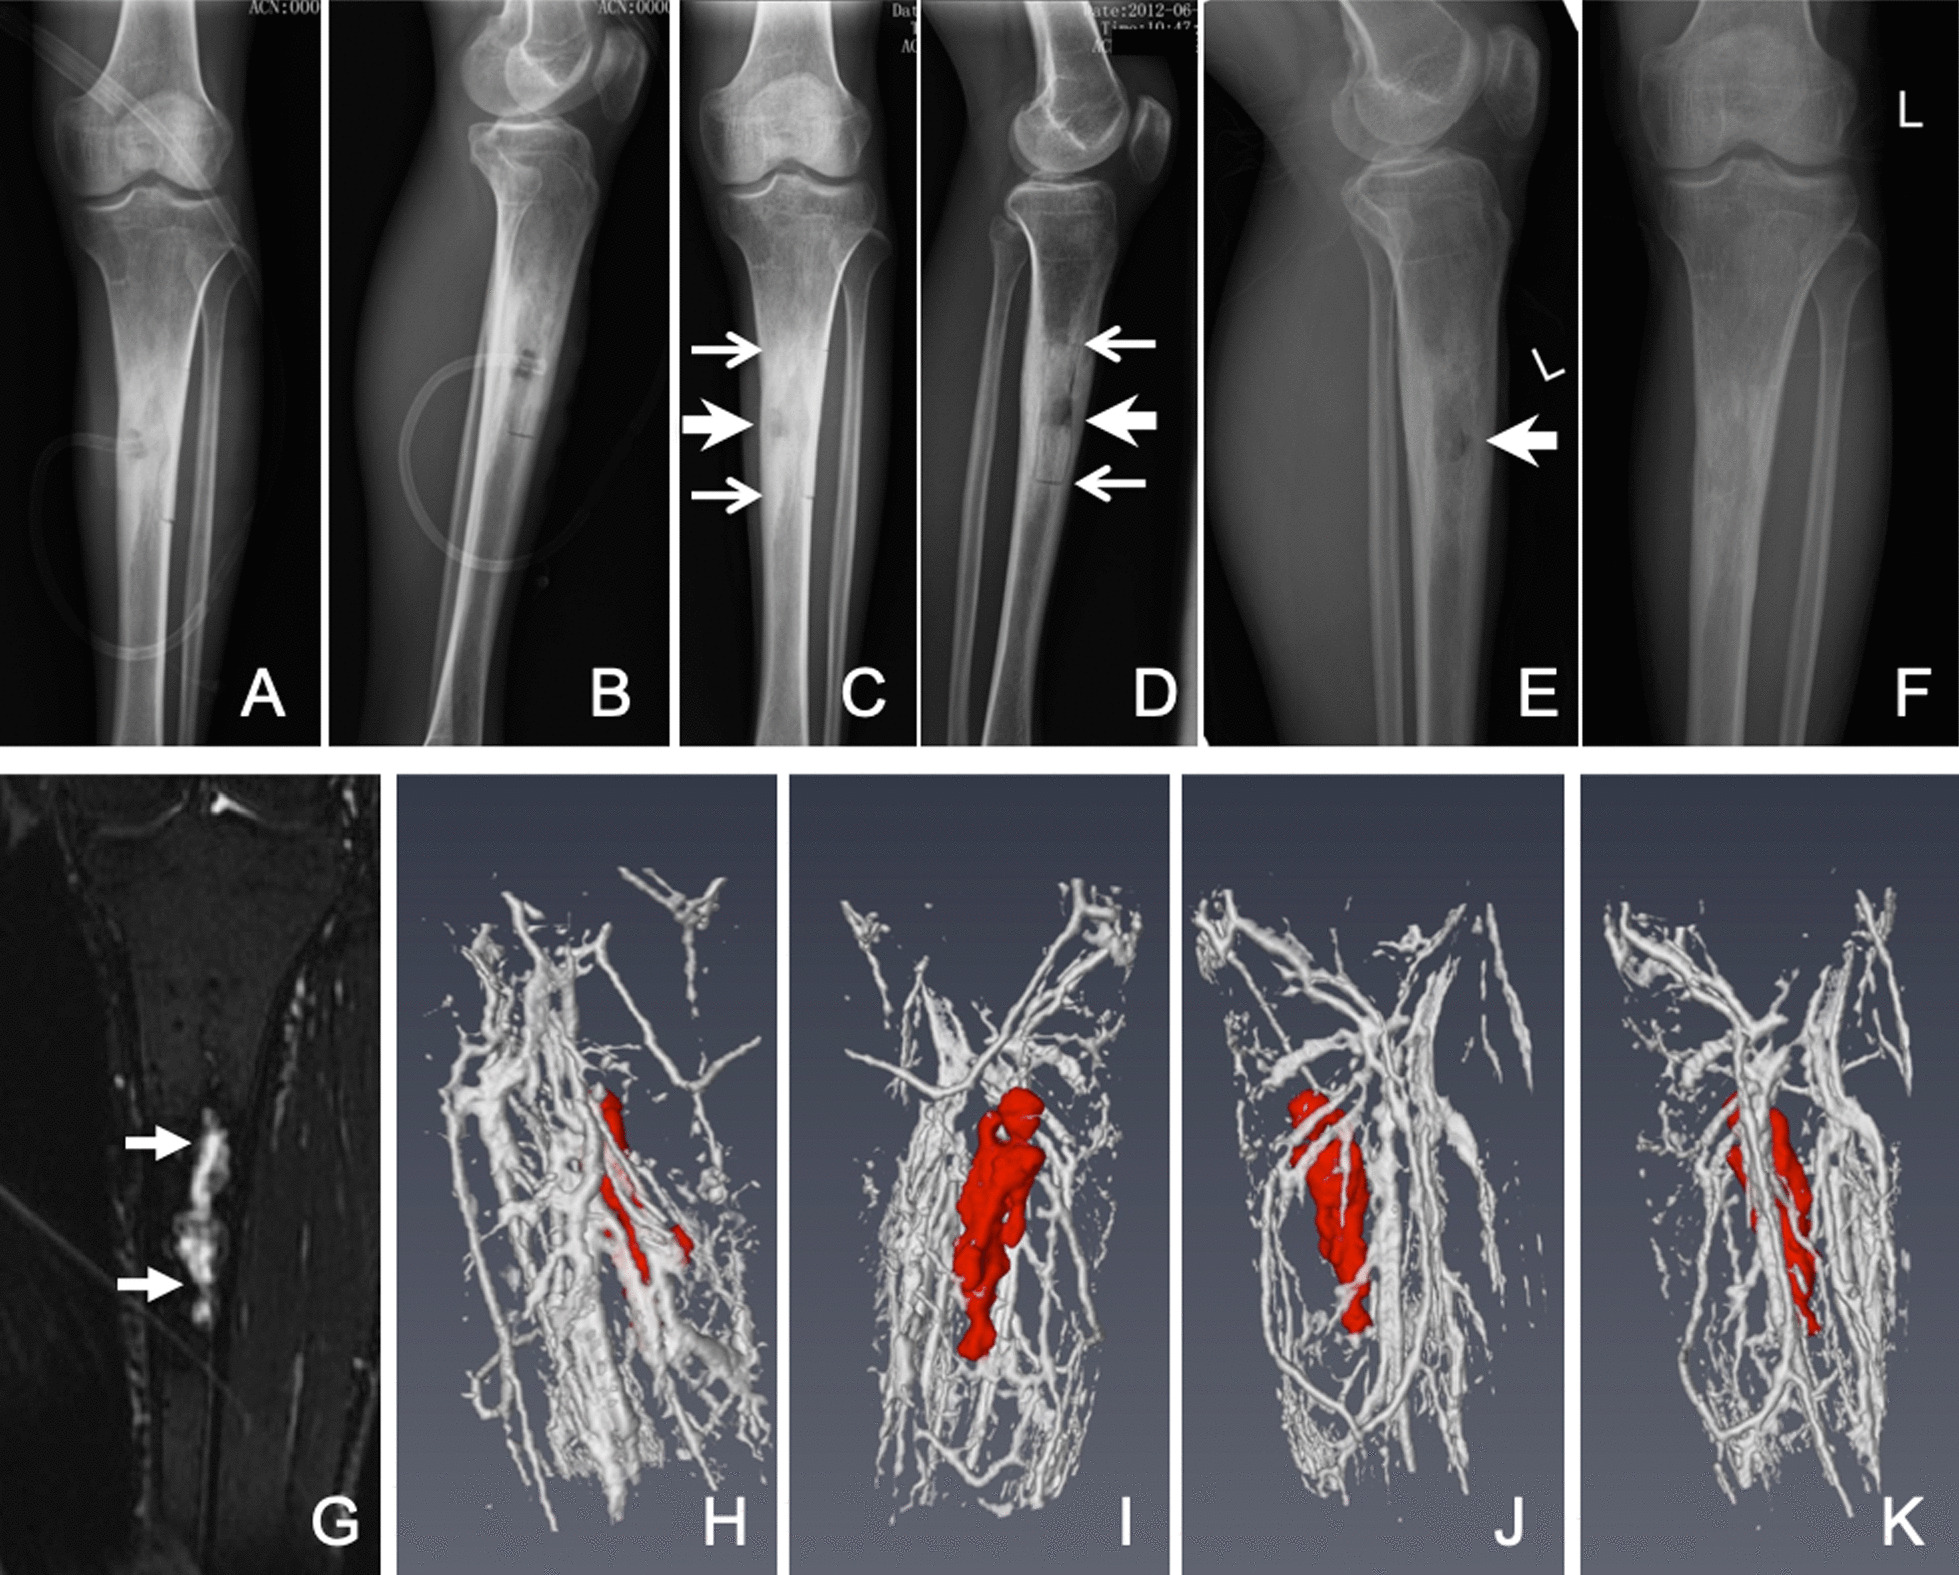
Long-term follow-up shown limited tibial fistulous tract still remaining. The post operation follow-up were performed by plain radiography on May 25th (A, B) and June 18th, 2012 (C, D); and April 16th, 2018 (E, F), and MRI on April 24th, 2018 (G–K). There is an open window for decompression drainage on the left tibial anteromedial side (A–D) which gradually healed and recanalization of the medullary cavity (E, F). Although the bone infection lesions were not able to completely removed (G: white arrows, red segmentations in H–K), the abundant blood supply around the lesions limited the spread of the infection lesions to the surrounding shown in MRI 3D reconstruction (H–K)
